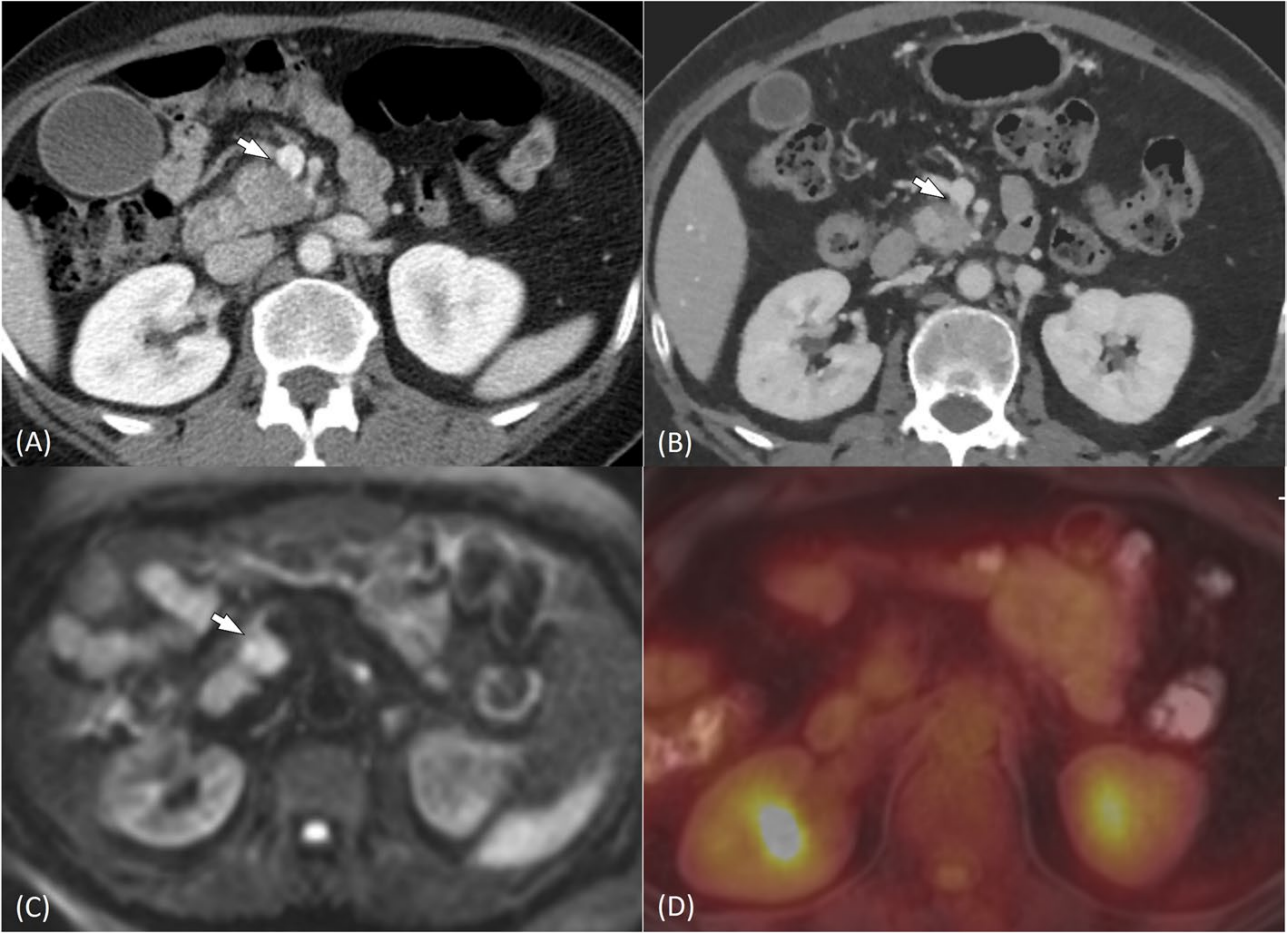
A 54-year-old woman with pancreatic cancer. Axial portal venous phase image of the baseline contrast-enhanced computed tomography (CECT) scan (A) demonstrates a 3 cm-sized pancreatic head cancer, contacting the superior mesenteric vein with vein contour irregularity (arrow). On arterial phase image of post-neoadjuvant therapy (NAT) CT scan (B), the size of pancreatic cancer decreased to 1.7 cm, but contour irregularity of the superior mesenteric vein was still noted (arrow). CECT resectability score was 3 (indeterminate resectability) according to the three reviewers in consensus. Diffusion restriction was seen at the tumor-vessel contact on diffusion-weighted imaging (DWI) (arrow) (C) and fluorodexyglucose (FDG) avidity at tumor-vessel contact was not seen on. ^18^F-fluorodexyglucose (FDG)-positron emission tomography (PET) (D) of PET/MRI. Carbohydrate antigen level (CA) 19–9 was 245U/mL at initial diagnosis, which reduced to 30 U/mL after NAT. The reviewers assigned resectability score 3 on CT plus PET/MRI set and score 4 on CT plus PET plus CA 19–9. The patient underwent Whipple’s surgery, and pathologic analysis showed College of American Pathologists grade 2 (moderate response) with ypT0N0
